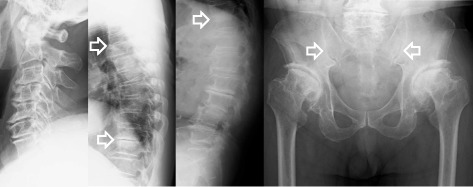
X-ray revealed T3/4 and T10/11 intervertebral disc space narrowing and anterior spondylolisthesis and no involvement of the sacroiliac joints (*white outline arrows*)
